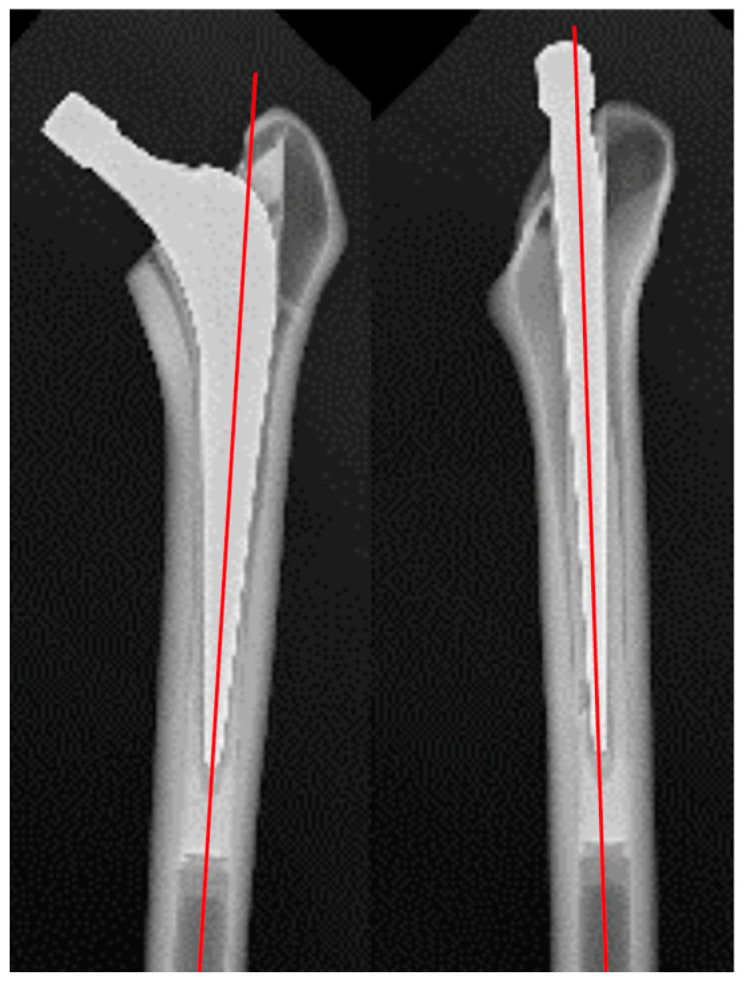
Representative anteroposterior and lateral plain radiographs showing that the alignment was within ±1 degree of the intended position.
